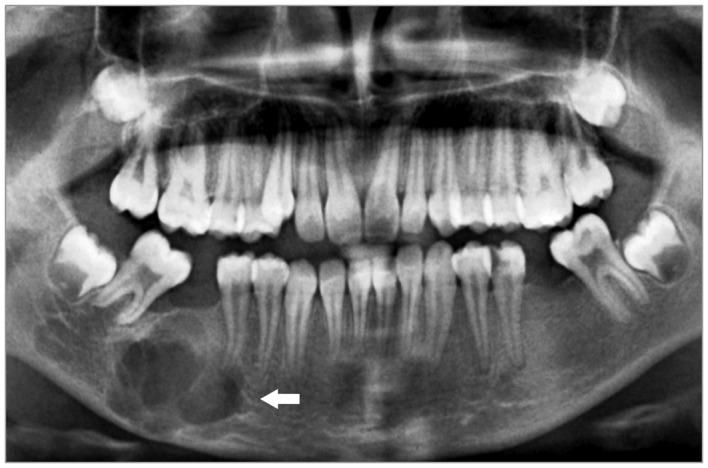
Radiograph indicating the 3.5 cm lesion (arrow) in the body of the right mandible.
